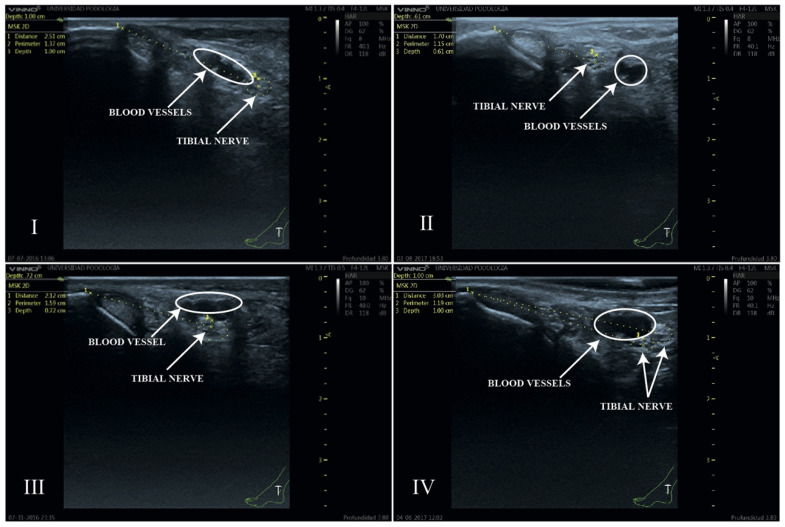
Ultrasound image of the four anatomical relationships between the TN and the PTA. Type I: TN posterior to PTA; Type II: TN anterior to PTA; Type III: TN lateral to PTA; Type IV: bifurcated TN. The white circles indicate the anatomical position of the blood vessels. The location of the tibial nerve has been defined by the yellow dashed circle of its perimeter.
